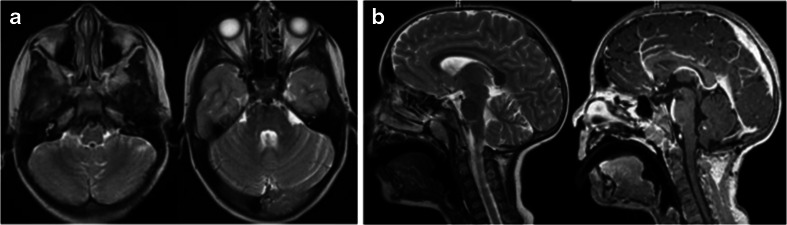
Case 3. Post-operative MRI (a T2-weight axial image, b T1-weight sagittal image after contrast) showing no evidence of residual or recurrent epidermoidCase 4. This is a 5-year-old boy who presented with increasing lethargy after a minor fall a week ago. On examination, he was noted to have speech dysarthria with decreased gag reflex on the left side. He has nystagmus at lateral and upward gaze, and a mild left-sided dysmetria. Head CT and subsequent MR showed a large mass in the left CMF without hydrocephalus (Fig. 12). Through a hockey stick incision and posterior fossa craniotomy and C1 arch resection, a large exophytic tumor was exposed in the cisterna magna and upper cervical subarachnoid space (Fig. 13). The tumor appeared to be originating from the posterolateral medulla oblongata dorsally to the root entrance of 9th and 10th nerves. It extended medially to the lateral wall of the fourth ventricle, rostrally into the cerebellar parenchyma and laterally and anteriorly into the CPA. A gross total resection was attained sparing all visible cranial nerves of 7th to 11th. The pathology showed a juvenile pilocytic astrocytoma (JPA) and it appeared to be originating from the inferior cerebellar peduncle. Postoperatively, he had dysphagia necessitating NG feeding. He had supplemental NG feeding for 2 years which was then stopped. He had to turn his head to the left side to assist swallowing for big meals. He did not receive any adjuvant therapy for the tumor. Presently, 14 years after the resection, he attends college and his speech and swallowing were normal. The only neurological findings were a slight deviation of uvula and soft palate, minimal dysmetria of the left side, and left neurosensory hearing loss. The latest MRI showed no evidence of tumor (Fig. 14)
